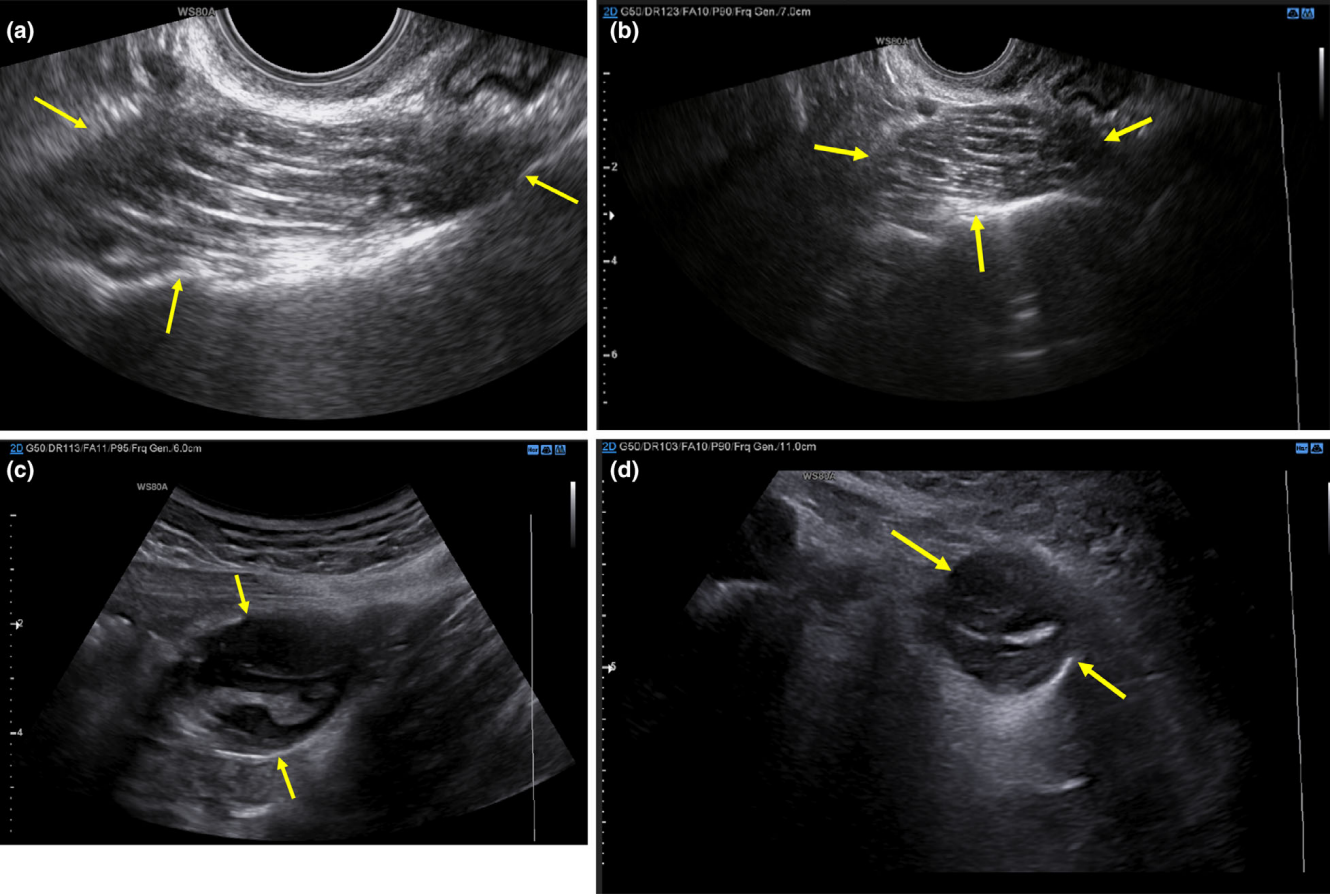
(a) Transvaginal ultrasound scan showing the appearance of the low‐grade appendiceal mucinous neoplasm at 8 weeks of pregnancy. (b) Transvaginal ultrasound scan in transverse showing the appearance of the low‐grade appendiceal mucinous neoplasm at 8 weeks of pregnancy. (c) Transabdominal (TA) ultrasound scan showing the appearance of the low‐grade appendiceal mucinous neoplasm (LAMN) at 8 weeks of pregnancy. The lower resolution from the lower frequency of TA shows a different appearance of the LAMN compared with the transvaginal images. (d) Transabdominal ultrasound scan in transverse section showing the appearance of the low‐grade appendiceal mucinous neoplasm at 8 weeks of pregnancy.
